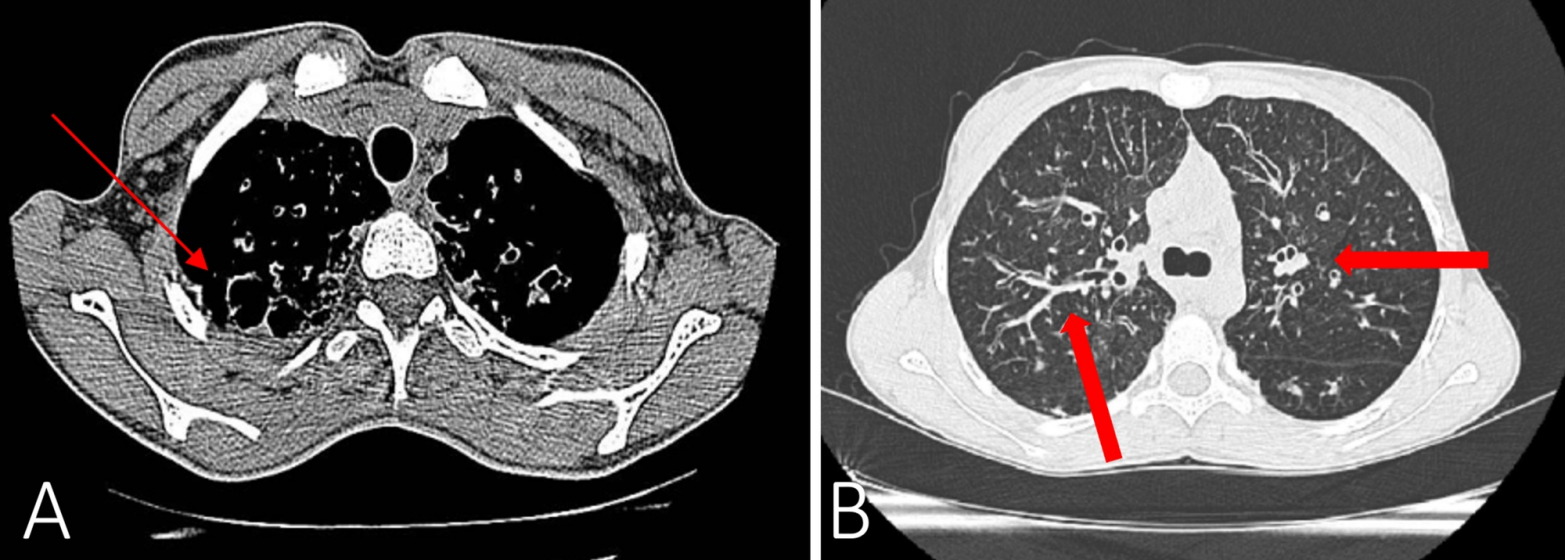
Chest CT of the apical lung field (A) showing the formation of cystic dilations on the right side (thin arrow) and of the middle lung field (B) showing signet-ring sign and cylindrical bronchiectasis (thick arrows)
